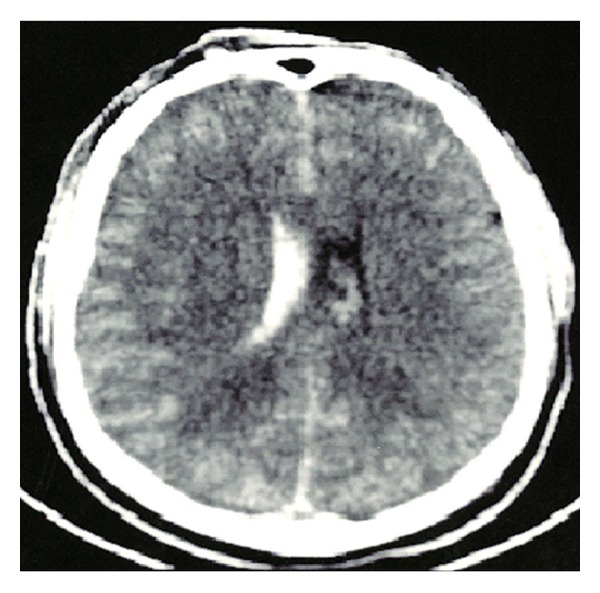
Postoperative axial CT scan demonstrates residual contusions in the right frontal lobe and in particular the presence of blood in the ventricular system.
